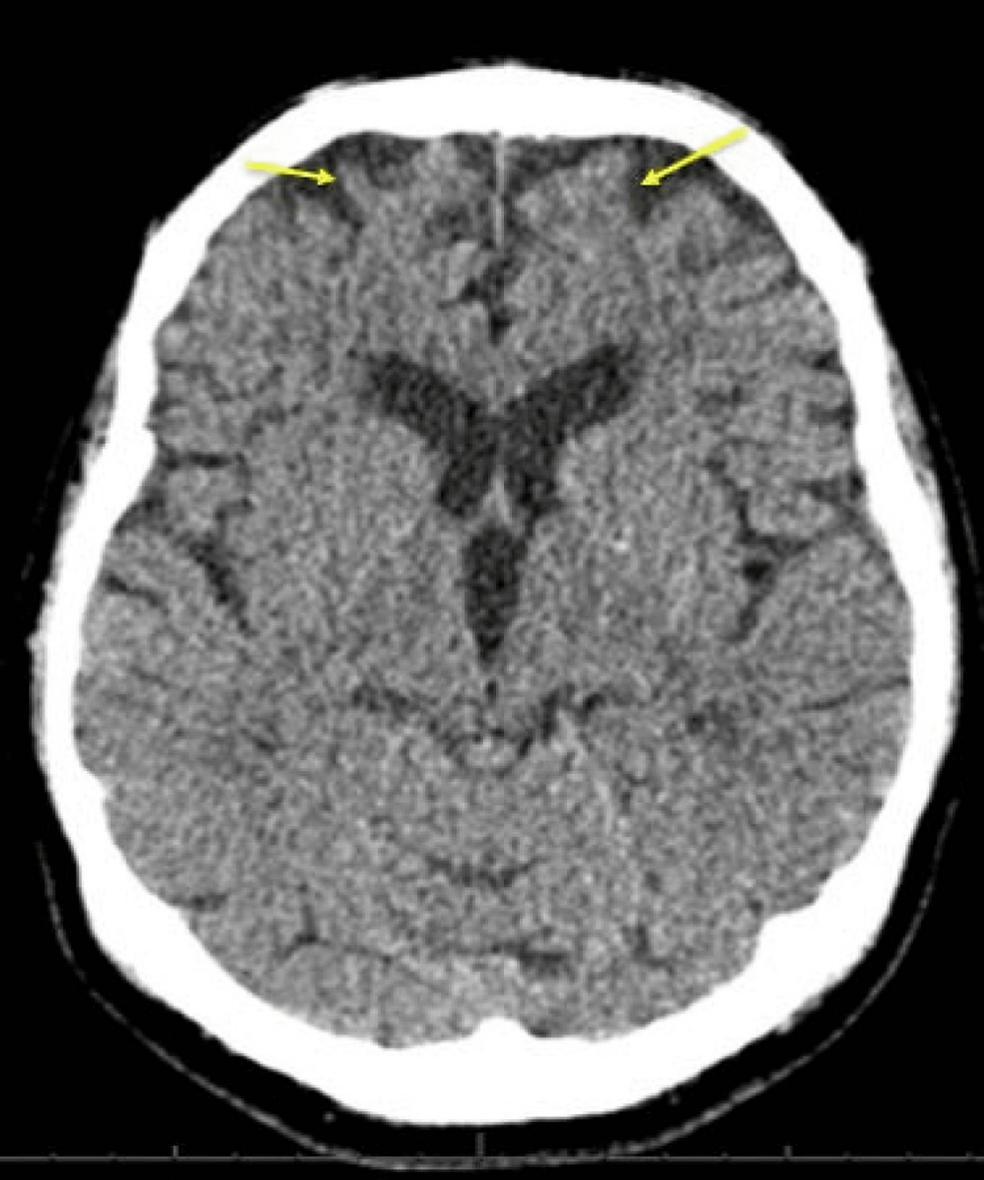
Non-contrast computed tomography of the head with nil acute findings. Normal ventricles and basal cisterns. Some atrophy of the frontal lobes appeared advanced for the patient’s age (yellow arrows).
